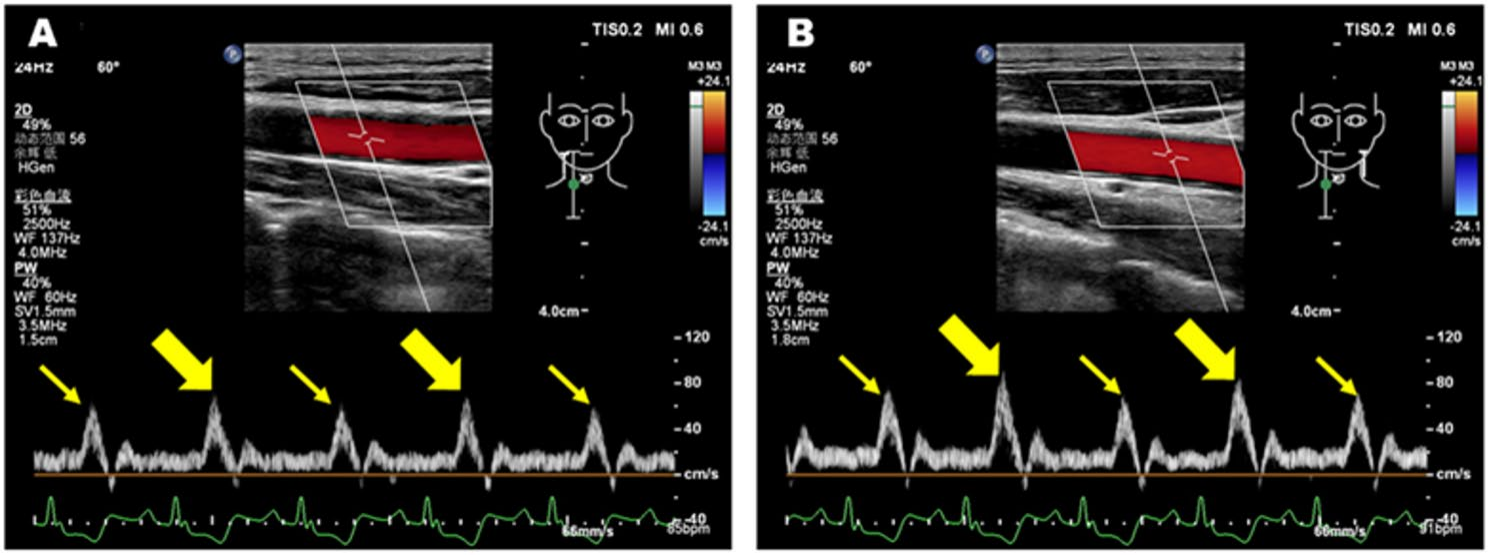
Doppler interrogation revealed marked beat-to-beat alterations in the bilateral carotid arteries of a patient with pulsus alternans. A Strong beat (bold yellow arrow) and weak beat (thin yellow arrow) of the right carotid artery. B Strong beat (bold yellow arrow) and weak beat (thin yellow arrow) of the left carotid artery
